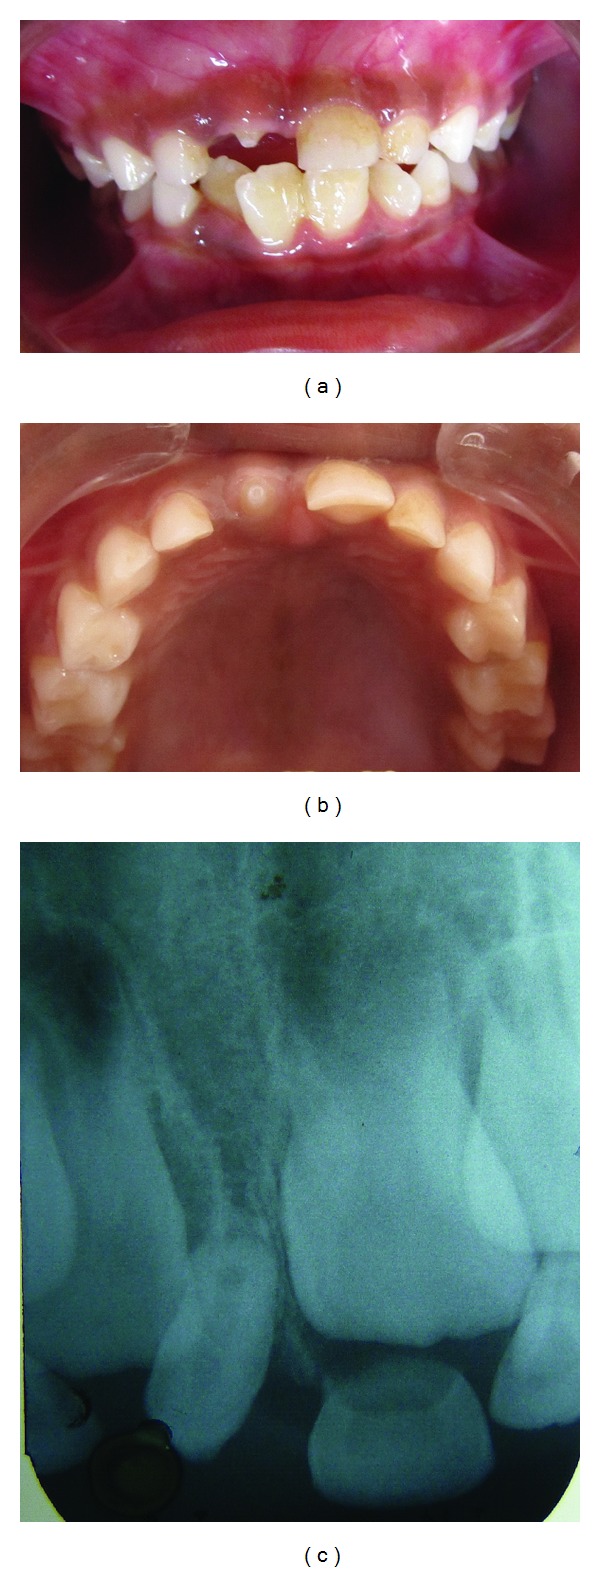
(a) Showing erupting mesiodens in place of central incisor, (b) showing occlusal view of mesiodens, and (c) radiograph showing mesiodens displacing permanent central incisor.
